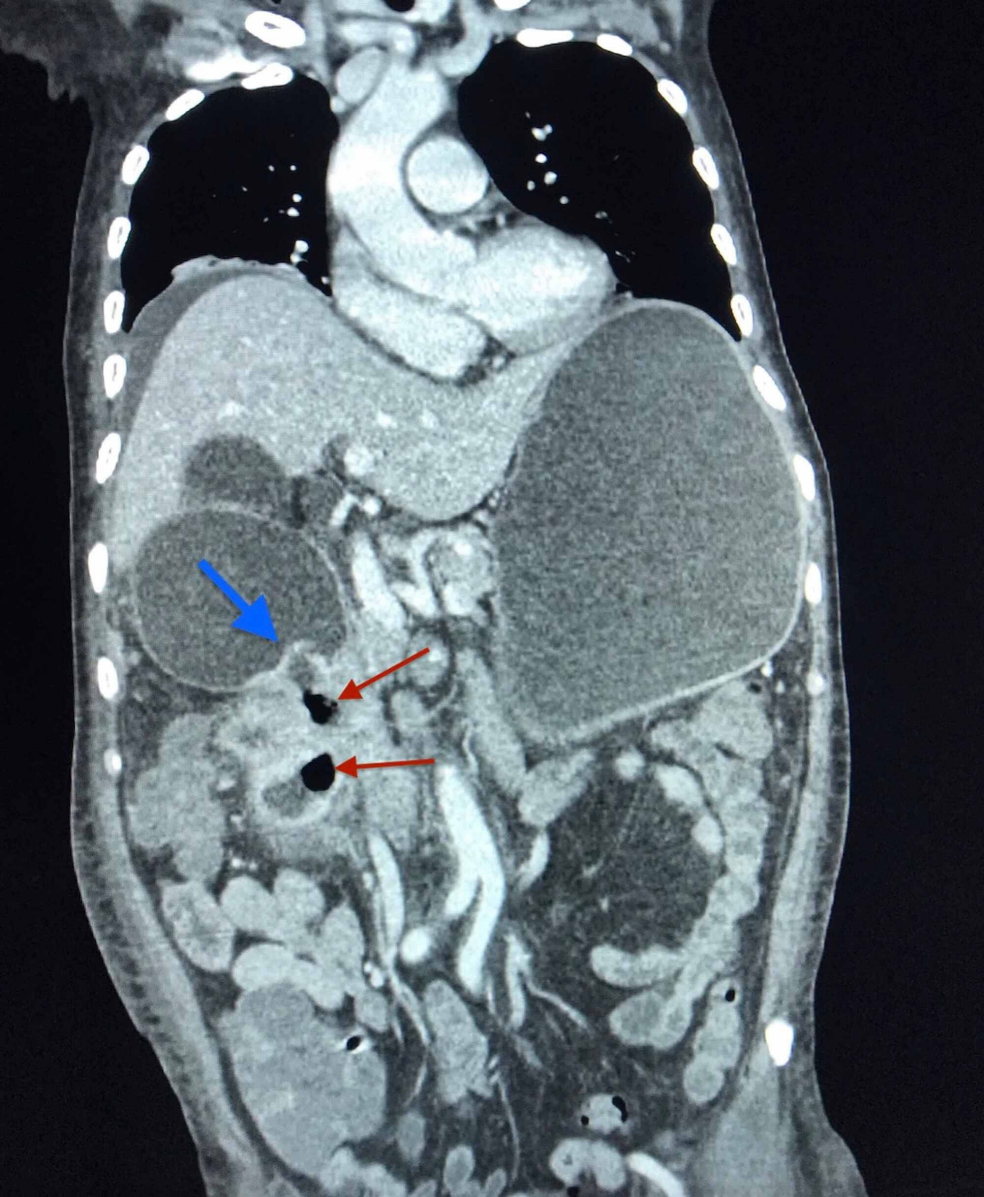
Pyelogastric fistula visualized connecting upper renal calculi with gastric antrum on CT of abdomen, coronal plane Fistula is indicated by the blue arrow. Locules of air are indicated by the red arrows.
